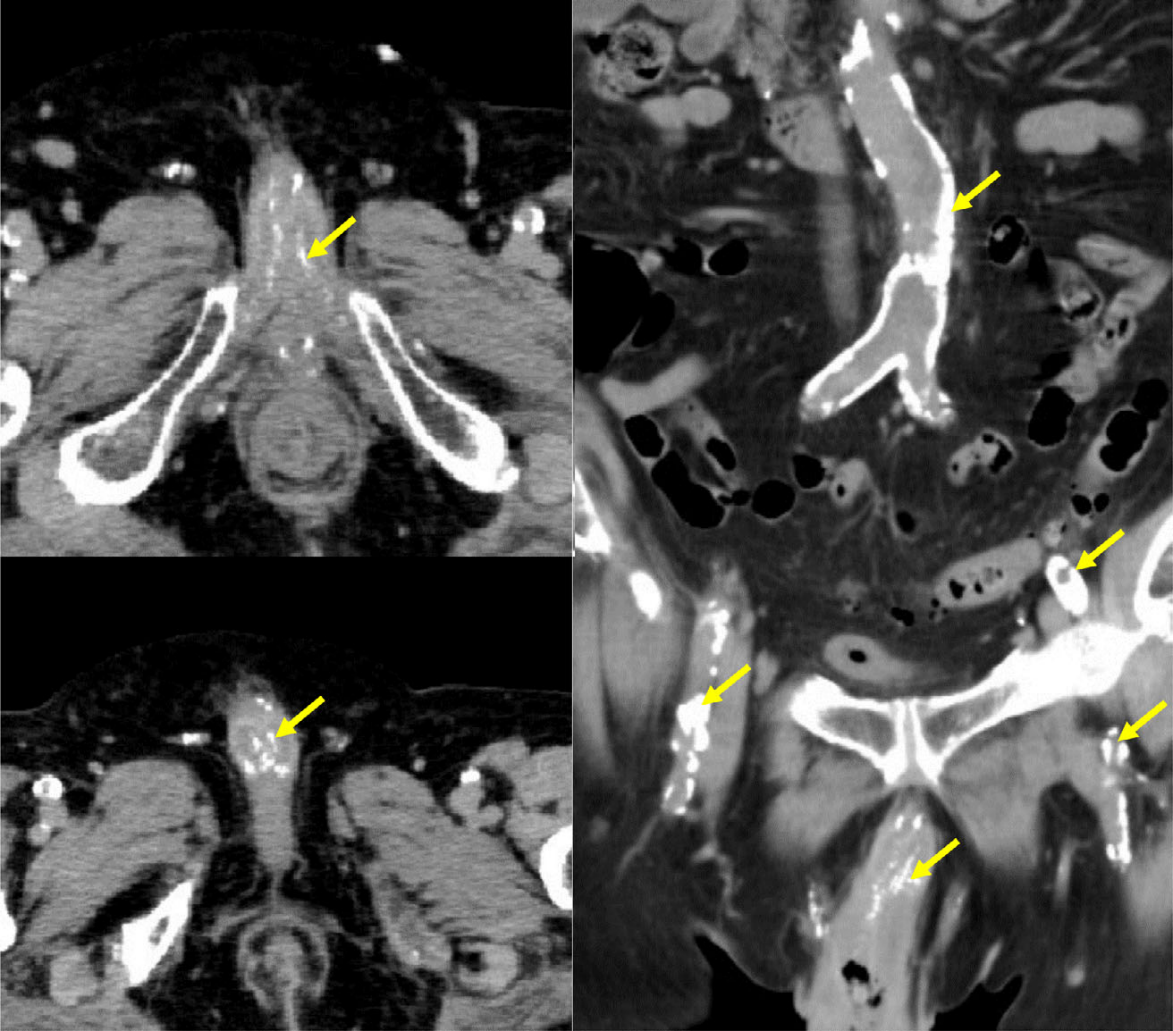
Plain computed tomography. Marked vascular calcification was observed. Aorta, iliac artery and penis blood vessel (arrows).
